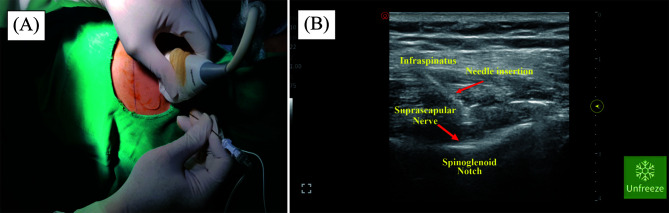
The suprascapular nerve at the supraglenoid notch using USG. The operator stands behind the patient, parallel to the ultrasound monitor, to achieve an ergonomic position. The ultrasound monitor is placed in front of the patient. The patient is in a lateral decubitus position, with the affected palm against the nonaffected shoulder and padding to protect the prominent area of the body. A sterile technique was used to prepare and clean the affected shoulder. The injection site for the suprascapular nerve block at the spinoglenoid notch is 2 cm below the spine scapula (A). An echogenic 23-G (3.5 in) spinal needle is inserted from medial to lateral (B). Injection (20 mg triamcinolone acetonide, 4 mL 2% lidocaine, and 4 mL 0.5% bupivacaine as a cocktail) was given and waited for 1–2 min.
